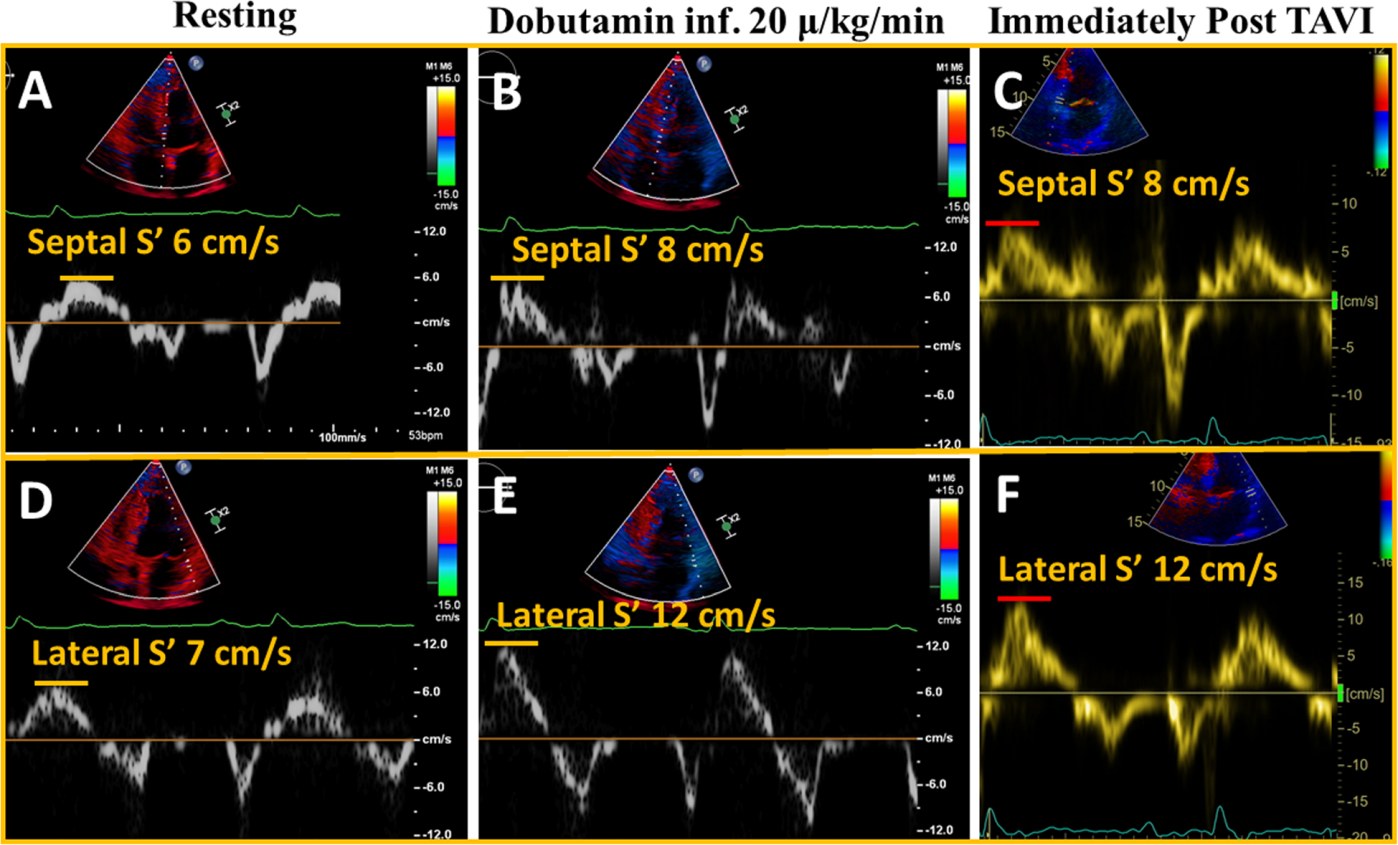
Septal and lateral S′ at rest (a, d), peak dobutamine stress echocardiography (b, e) and immediately after TAVI (c, f)
